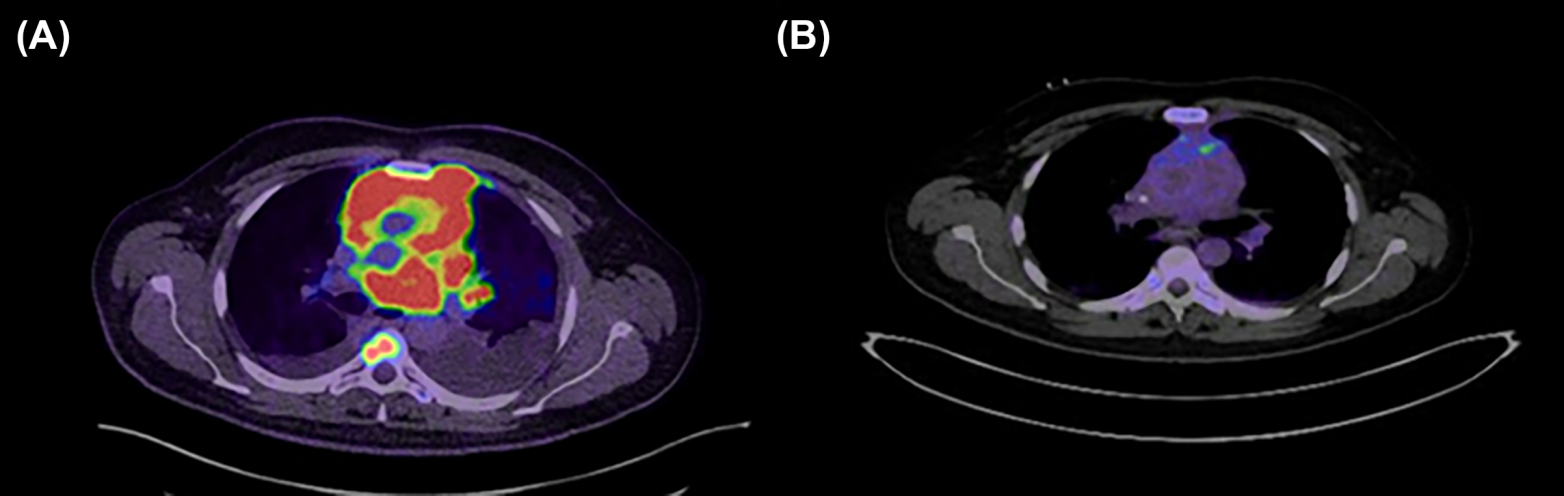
(A) An intense FDG avid mass was seen in the anterior mediastinum extending into the anterior chest wall and posteriorly to the mid/posterior mediastinum region. (B) Interval almost complete resolution of the intense FDG uptake that was previously seen mediastinal region, lower bilateral paratracheal, subcarinal, left hilar and right posterior mediastinum.
